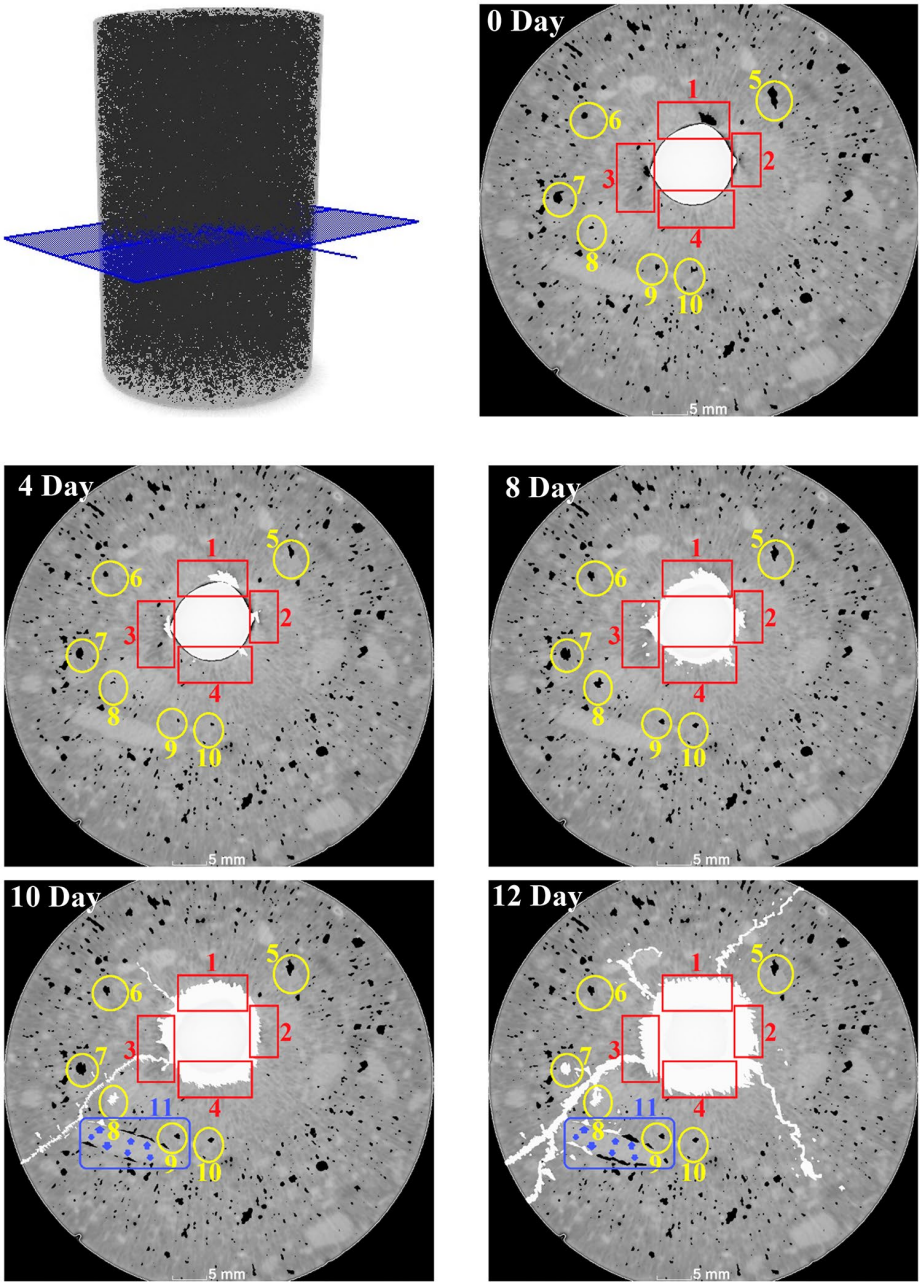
CT images of the pore structure and cracks in the vicinity of the reinforcing bar during various stages of accelerated corrosion.
